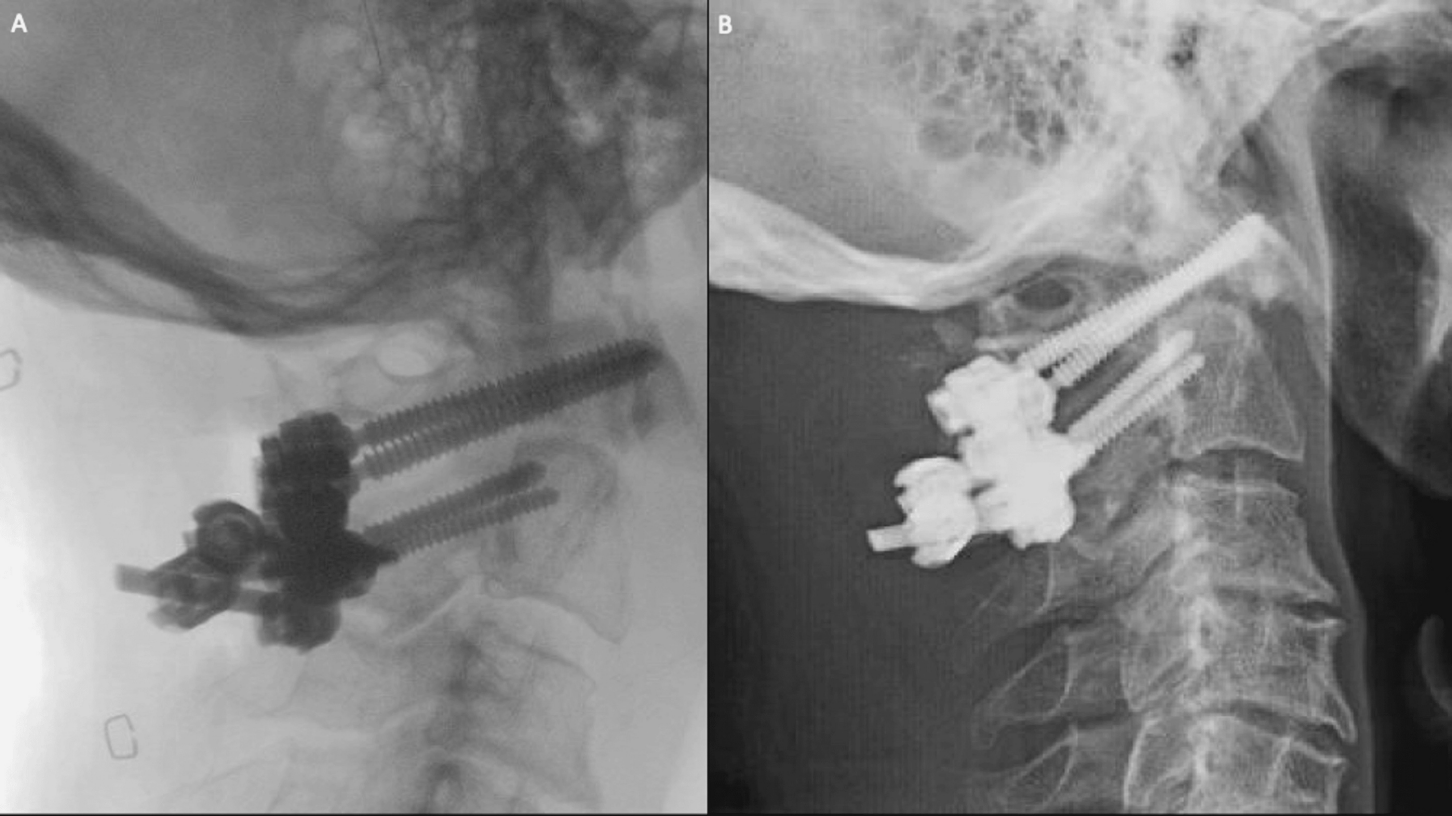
(A) Final intraoperative X-rays demonstrate improved atlantodental interval. (B) Eight-week postoperative X-rays showed no change in instrumentation, despite the patient’s non-compliance with external orthosis and Parkinsonian tremor.
